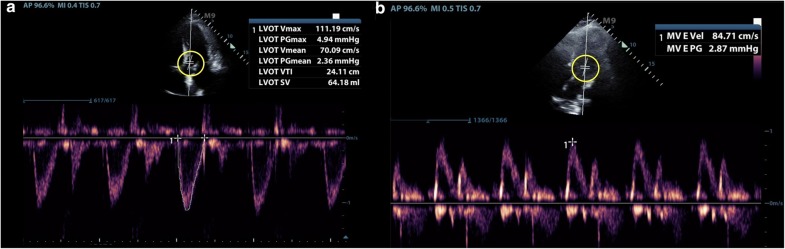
Angle correction (circles) in pulsed-wave Doppler on echocardiography, a practice that is not recommended and was performed in the study of Betcher et al. [1]. a Left ventricle outflow-tract flow; b transmitral flow. Alignment between the flow and the Doppler must be performed manually and thus requires an excellent technique, without electronic angle correction
